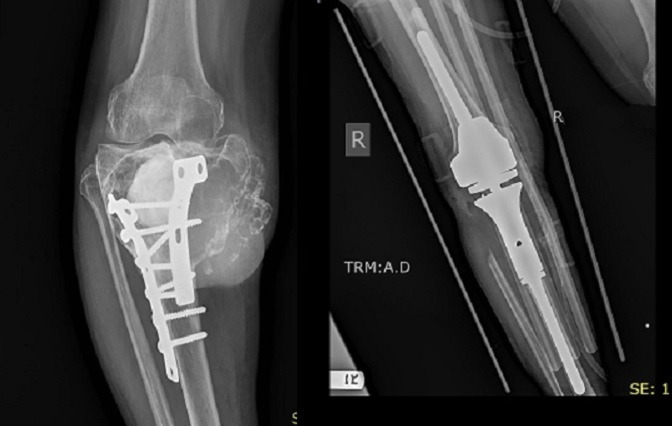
AP radiograph of the right knee preoperatively with recurrent giant cell tumor that was found to have undergone sarcomatous transformation. AP radiograph of the right knee after resection and reconstruction with a proximal tibial replacement.
